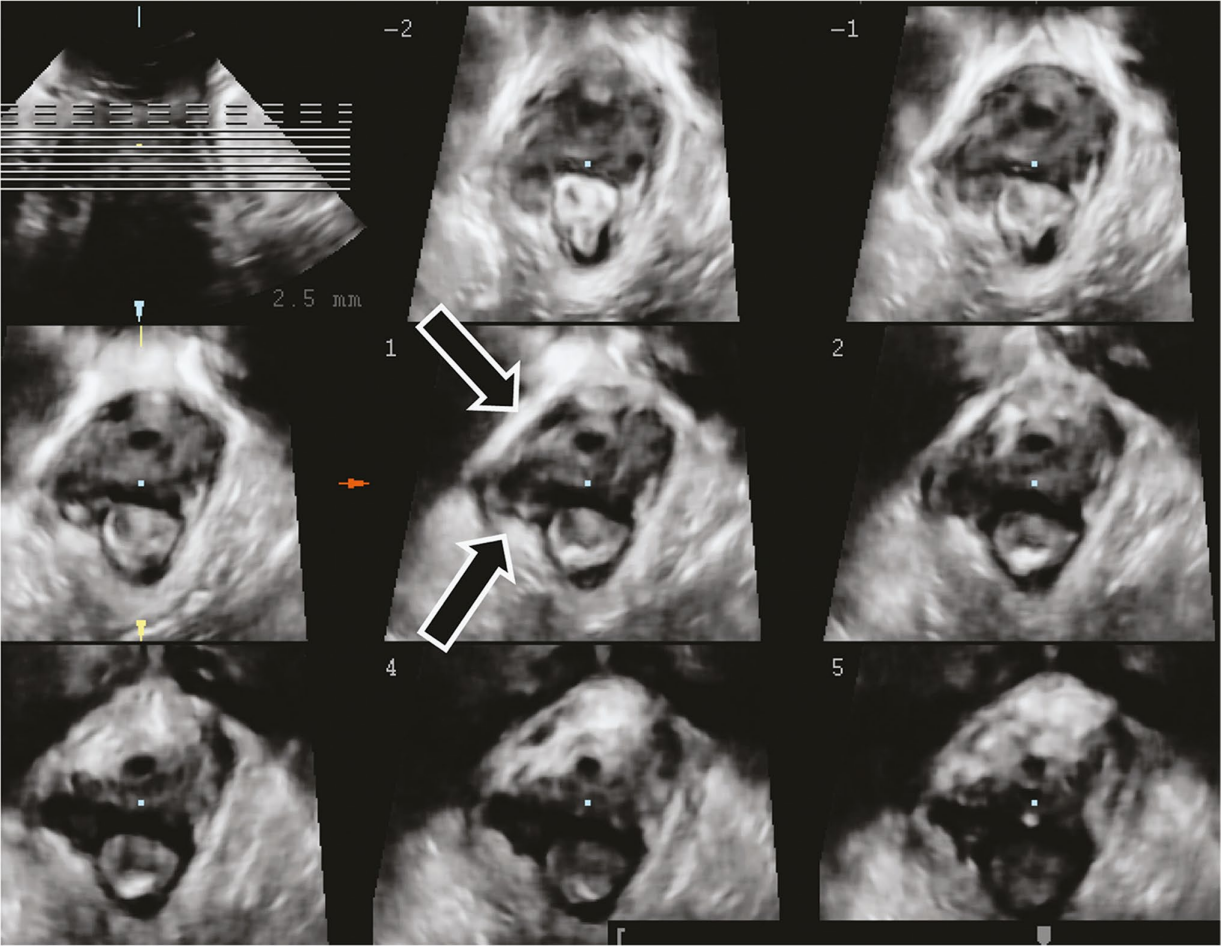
Ultrasound image of the pelvic floor in a woman following forceps vaginal delivery, showing avulsion of the levator plate from the right pubic ramus. Courtesy of Professor HP Dietz [49]. *Source:* Reproduced with permission of the Licensor through PLSclear
